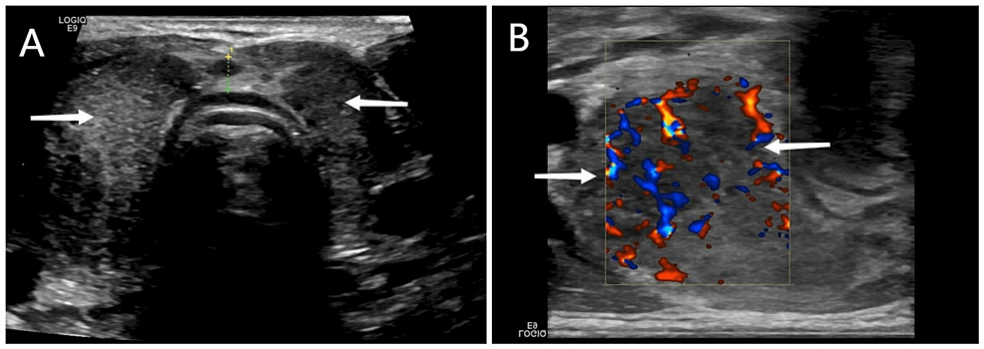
Ultrasound scan of the thyroid gland at three weeks from symptom onset. Enlarged thyroid gland with blurred margin (A), and relatively increased vascularization in ultrasound (B).
